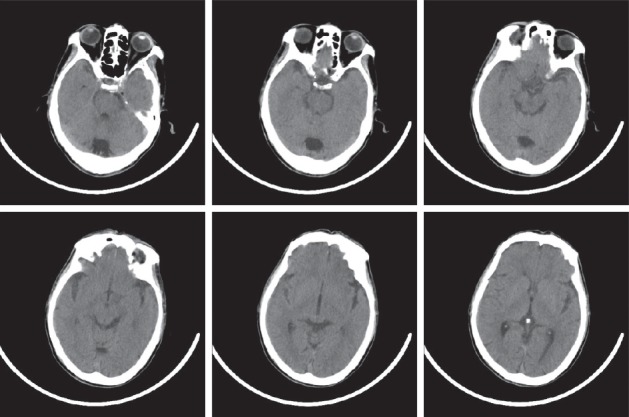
The CT image in July 28th. No blood could be seen.
